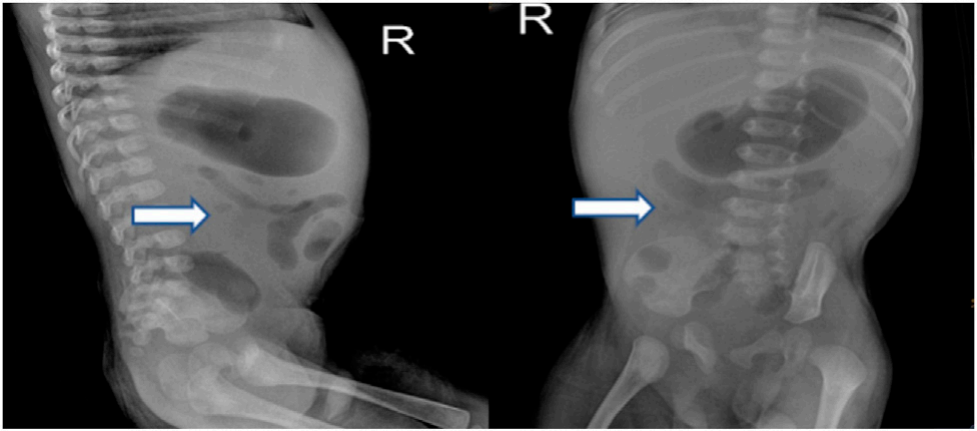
X-ray of the proband’s abdomen revealed stiffness in the intestines and low volume of intestinal gas.
